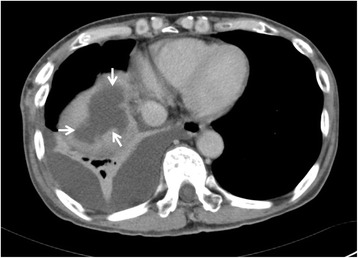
Computed tomography shows a low-density lesion (*arrows*) at the cut surface of the liver in the subphrenic space
